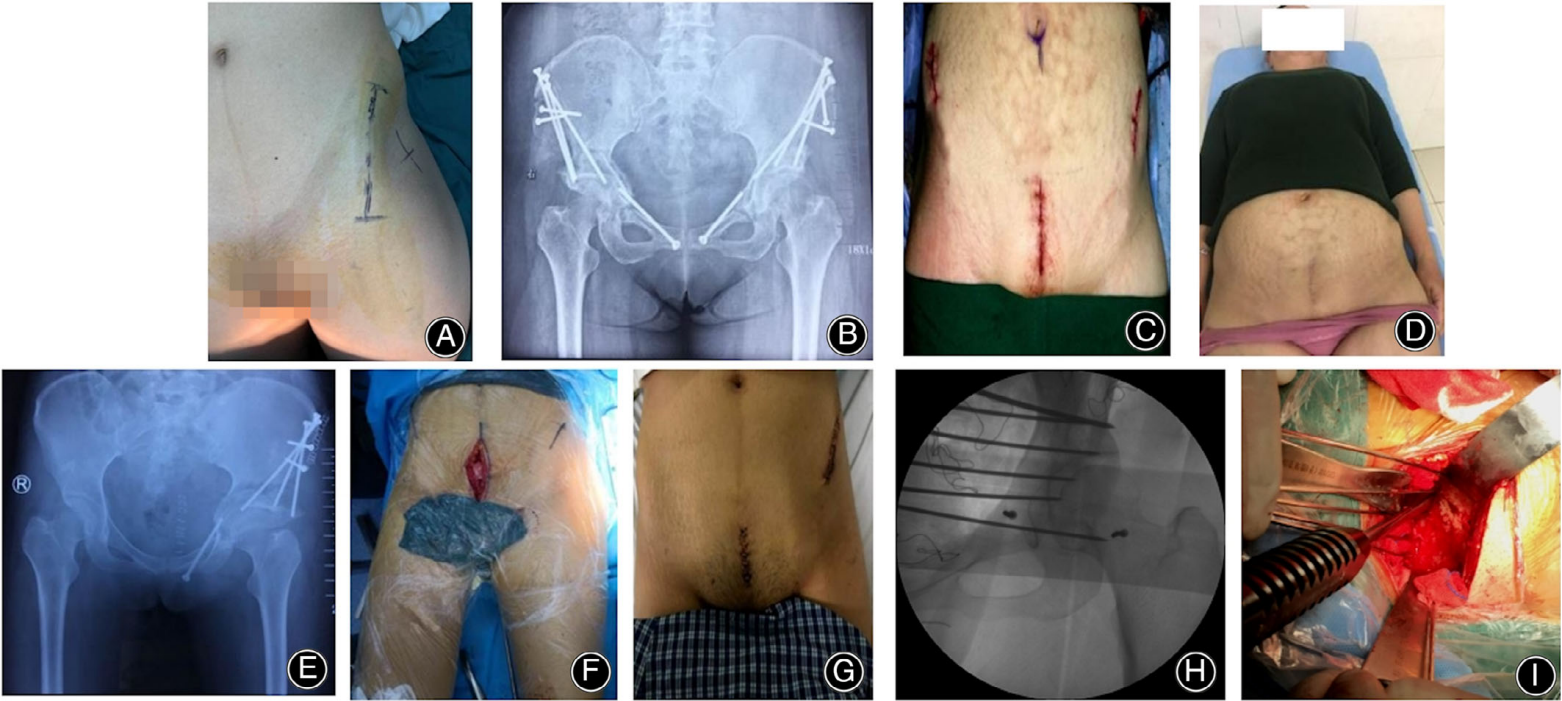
Two examples of patients with developmental dysplasia of the hip. (A) The incision of modified smith‐peterson approach. (B–D) A postoperative pelvic anteroposterior X‐ray and incision appearance of a patient with bilateral hip dysplasia using Stoppa combined with the iliac spine approach; (E–G) A postoperative anteroposterior pelvic radiograph of a patient with unilateral hip dysplasia using Stoppa combined with the iliac spine approach and the intraoperative and postoperative incision appearance. (H, I) The exposed quadrilateral area of the acetabulum during surgery.
